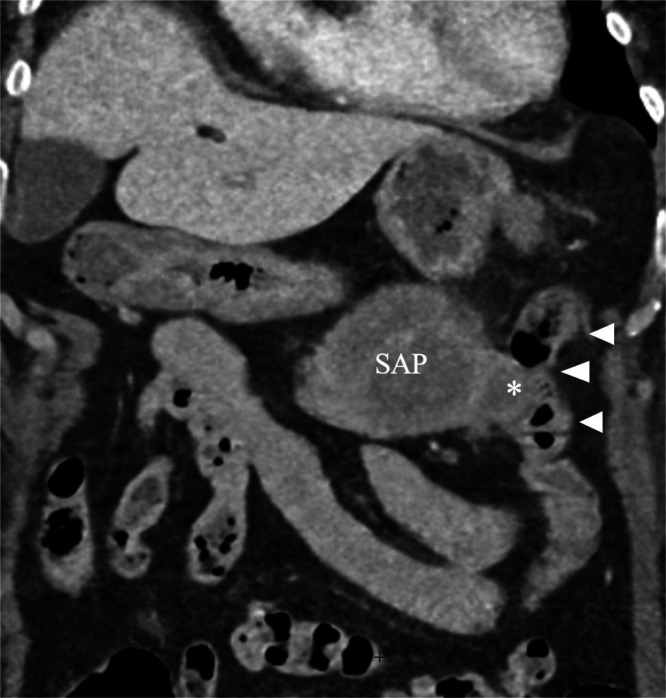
Contrast-enhanced CT, coronal MPR, showing a fistulous tract (asterisk) between the pseudoaneurysm (SAP) and the splenic flexure of the colon (arrowheads).
